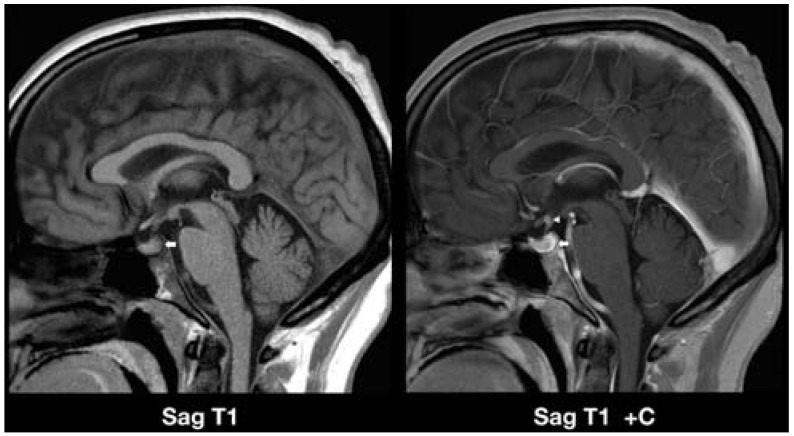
Leukemic infiltration of the neurohypophysis and pituitary stalk in a patient with myelodysplastic syndrome presenting with diabetes insipidus. The sagittal T1-weighted image without contrast administration (left image) demonstrates the absence of the neurohypophysis (arrow), confirming the diagnosis of diabetes insipidus. Normally, the neurohypophysis is identified as a bright signal spot on T1-weighted images in the posterior pituitary gland. The sagittal T1-weighted image after contrast administration (right image) reveals a 2-mm enhancing nodular lesion in the superior aspect of the pituitary stalk (arrowhead) and curvilinear enhancement along the posterior aspect of the pituitary gland in the expected region of the neurohypophysis (arrow). Sag, sagittal; C+, post-contrast.
